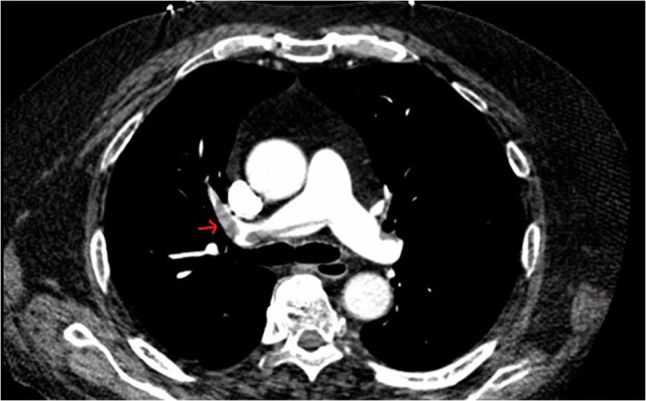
CT angiogram of the chest with bilateral pulmonary emboli in the left and right main pulmonary arteries.
